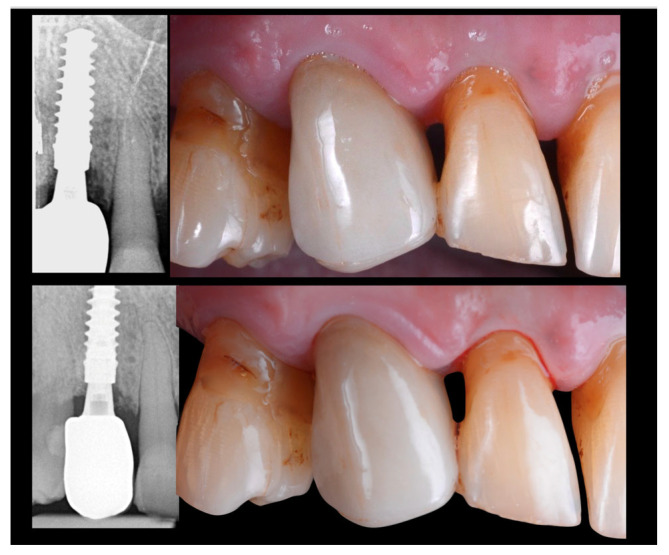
Clinical images and x-rays evaluation after the definitive crown installation (upper side) and after 2 years (lower side).
